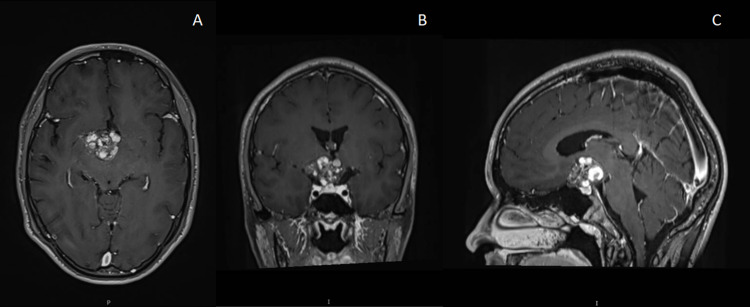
T1-weighted MRI at the time of arrival to our Institution. Rebleeding of the hypothalamic cavernous malformation is appreciated in different projections. A, Axial. B, Coronal. C, Sagittal.
